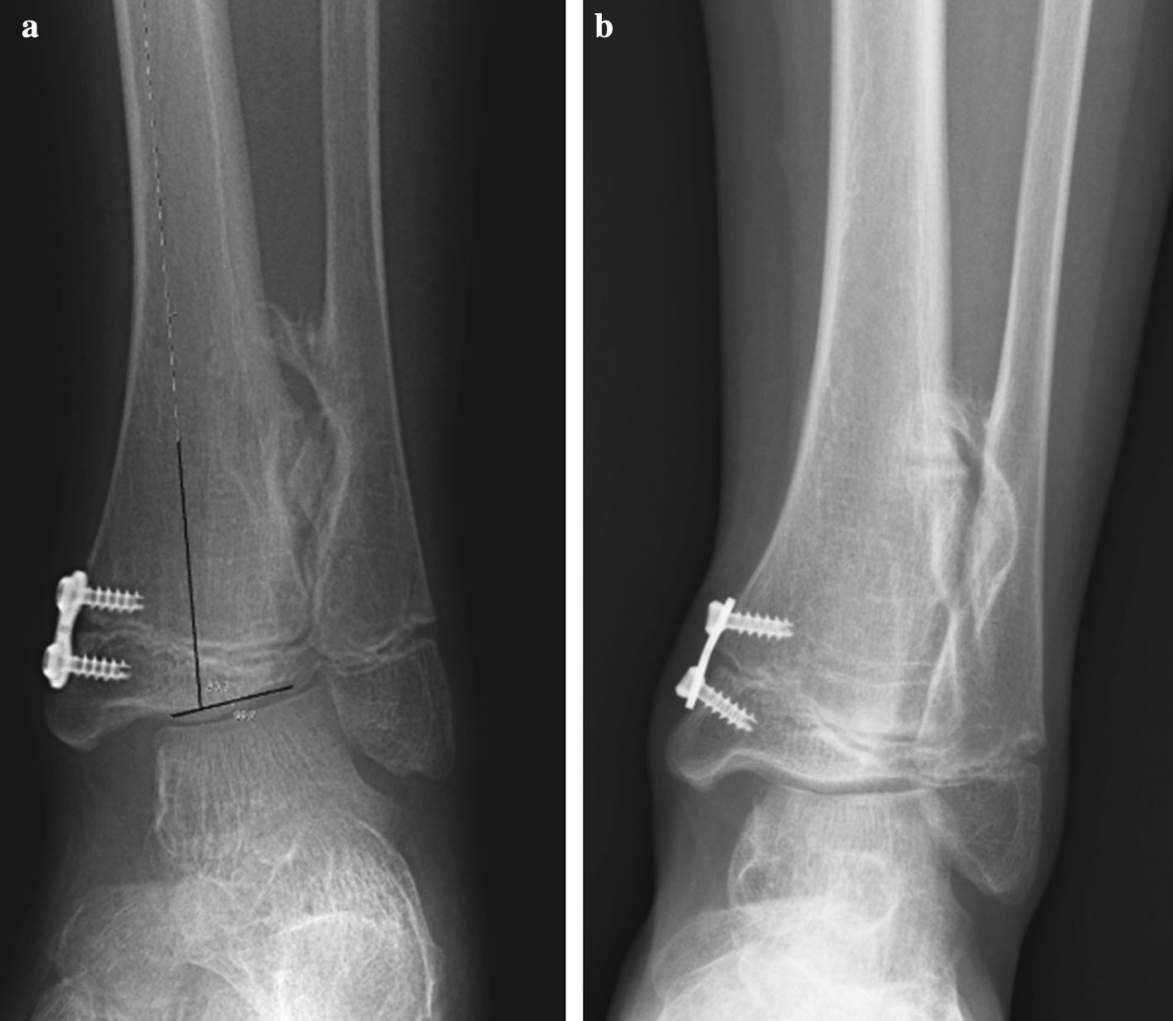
Radiograph obtained immediately following surgery (a) and later during follow-up (b)
